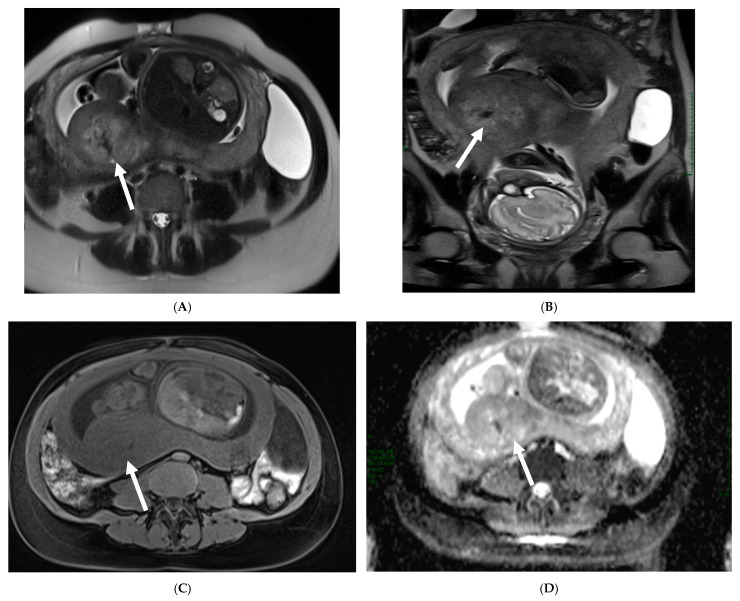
Placental chorioangioma in a patient at 26 weeks of gestational age and profuse vaginal bleeding. Coronal (A) and axial (B) T2-weighted HASTE and axial T1-weighted (C) images show an intrauterine rounded mass peripherally located and protruding from the fetal surface of the placenta (arrows). The mass is homogeneous and nearly isointense to the placenta on T1- and T2-weighted images, as well as on Diffusion Image (D) (arrows).
